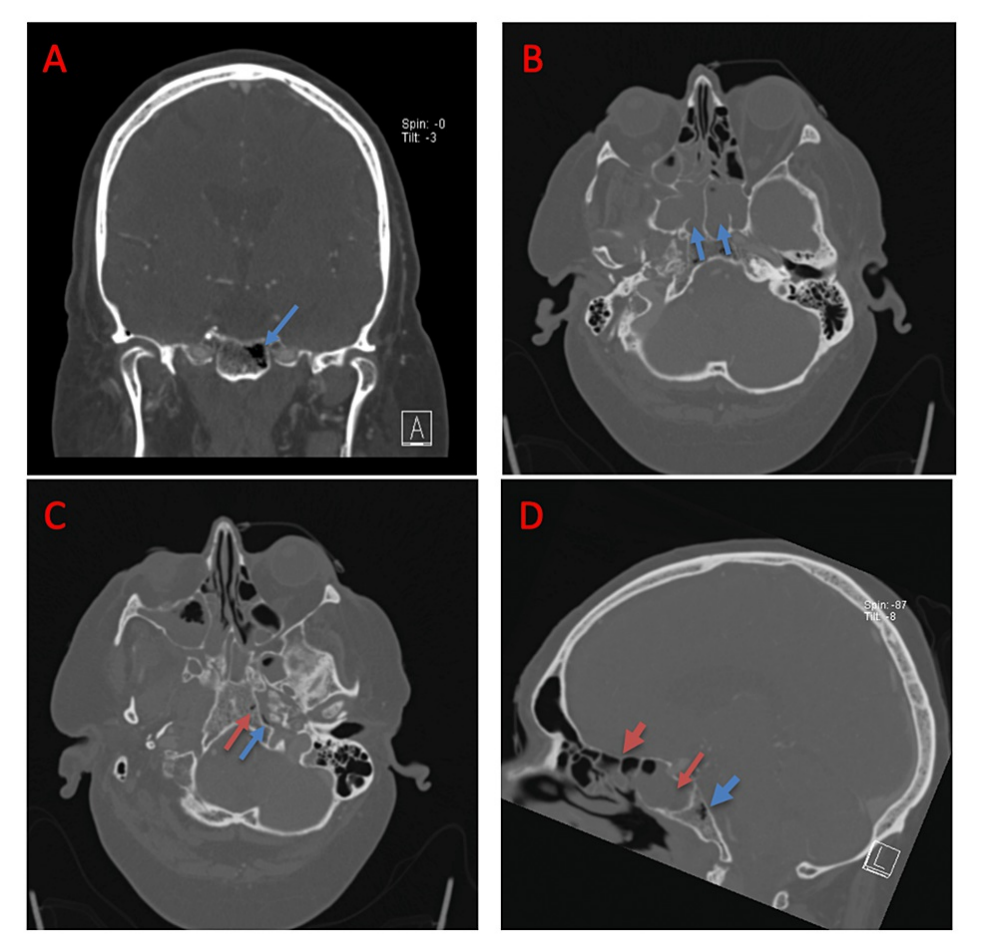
Computed tomography angiography of the head and neck. Head computed tomography angiography demonstrates the presence of gas within the clivus on the coronal section (A). The axial section demonstrates sinusitis and particularly pronounced sphenoid sinus disease (B). The axial section reveals the presence of gas within the clivus (red arrow) and the presence of curvilinear gas in the soft tissues anterolateral to the clivus (blue arrow) (C). The sagittal section (D) reveals patchy sinus opacification (red arrows) and redemonstrates gas within the clivus (blue arrow).
